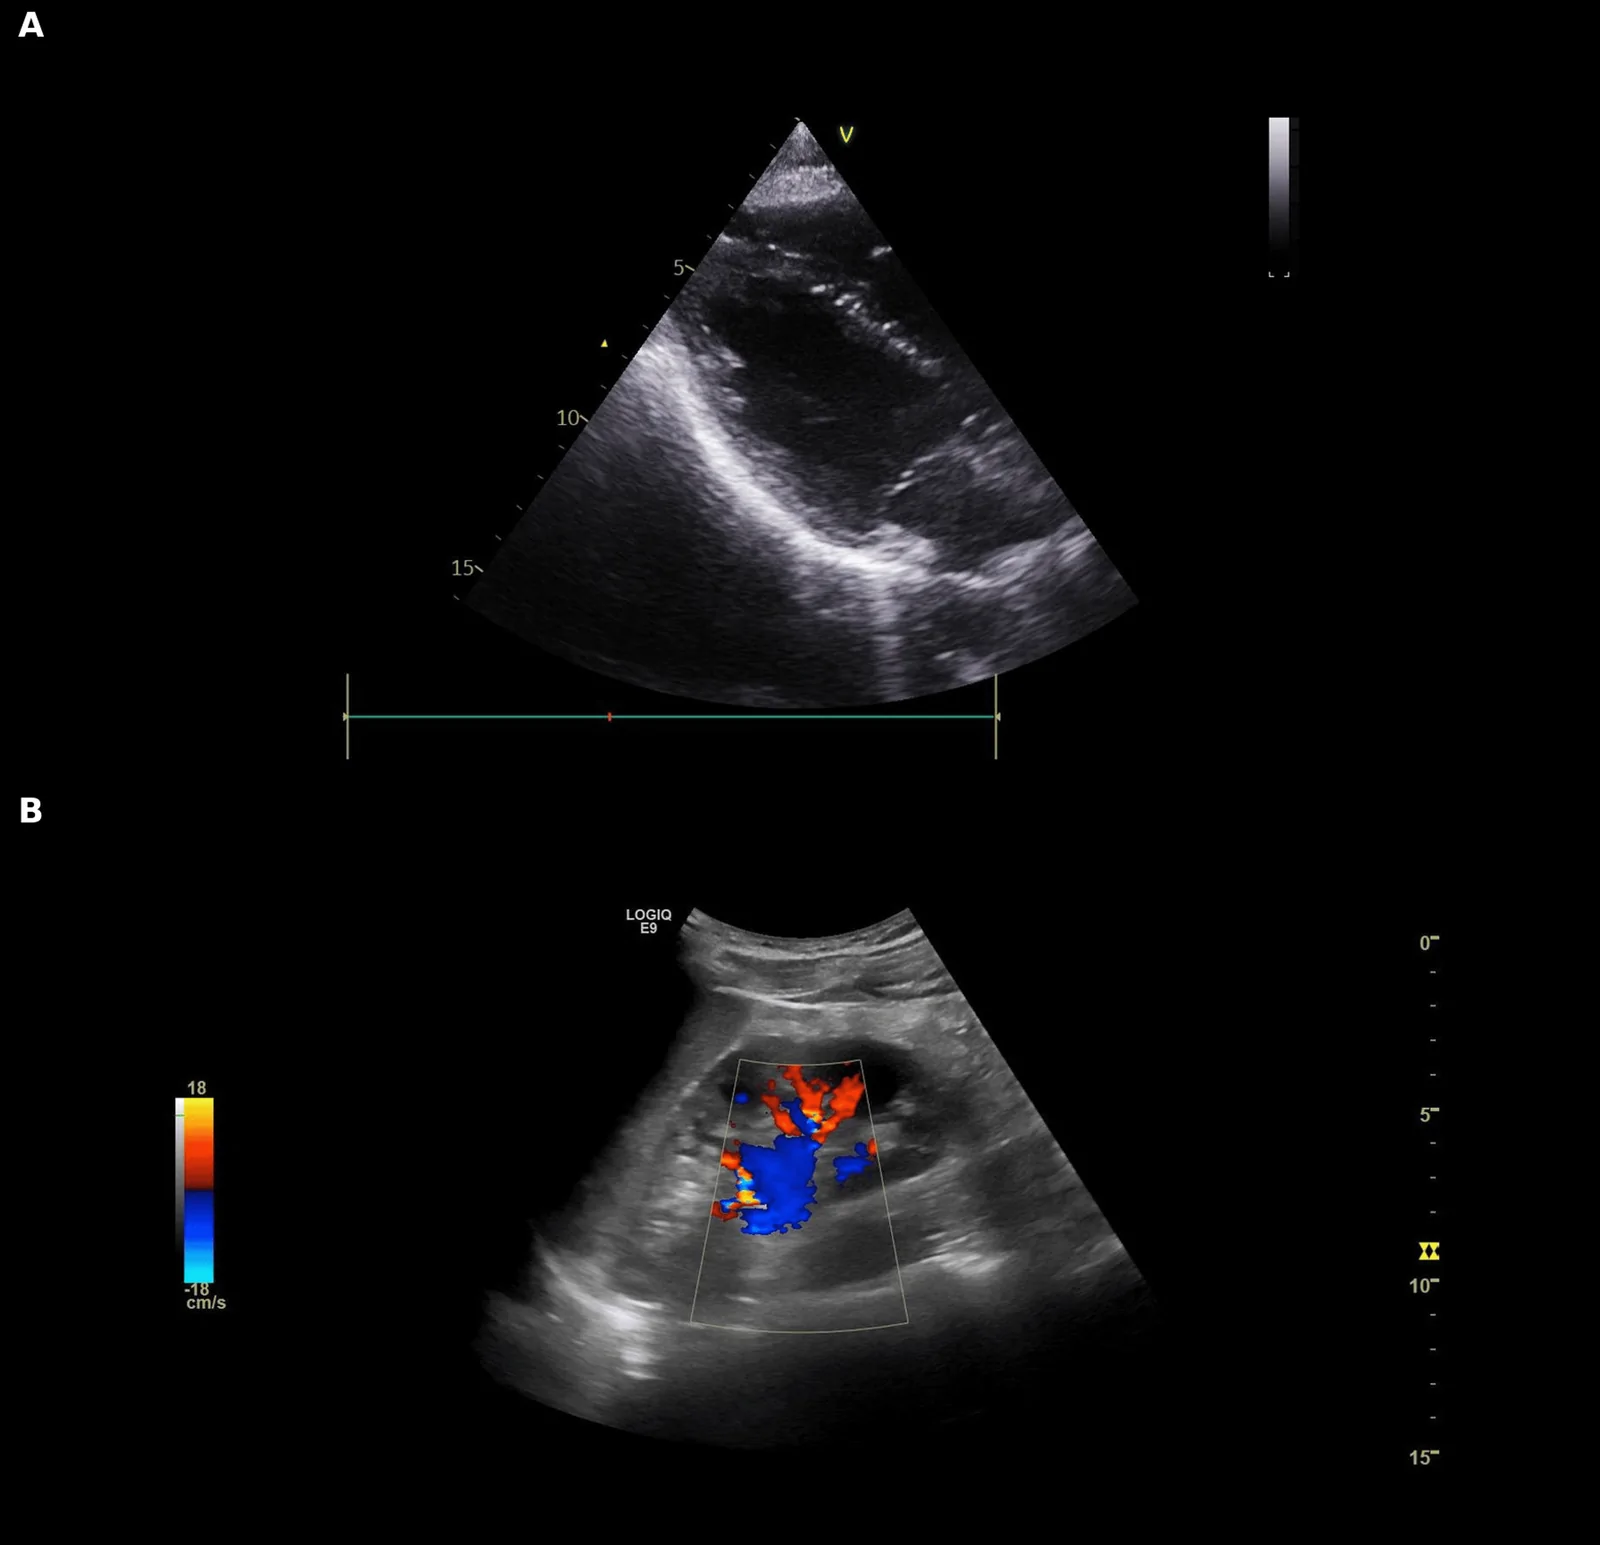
Right Renal Ultrasonography. (A) Grayscale image from the right renal examination demonstrating marked fluid distension of the pelvicalyceal system. (B) Color Doppler image from the same renal examination. The ultrasound report described severe right hydronephrosis with cortical thinning and no ureteric dilatation.
